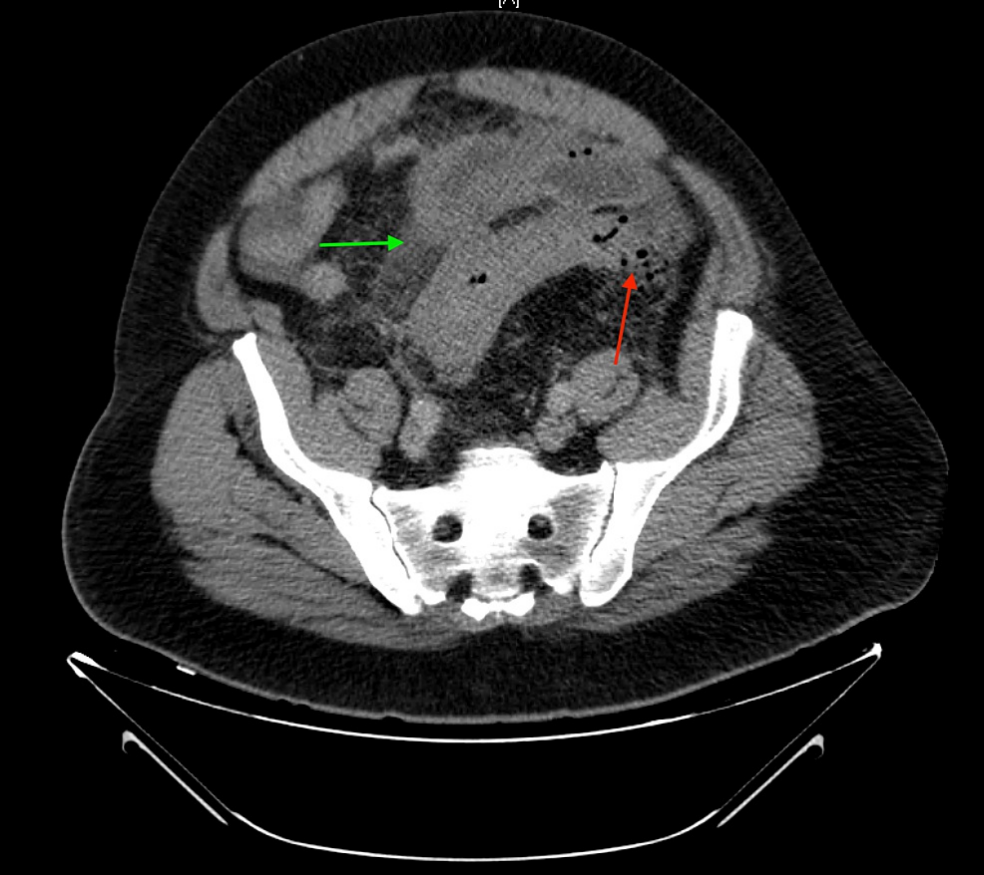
Transverse section of CT abdomen and pelvis with IV contrast from the subsequent emergency department visit, demonstrating sigmoid colonic wall thickening, fat stranding consistent with diverticulitis (red arrow), and the development of an encapsulated fluid collection consistent with abscess formation (green arrow)
